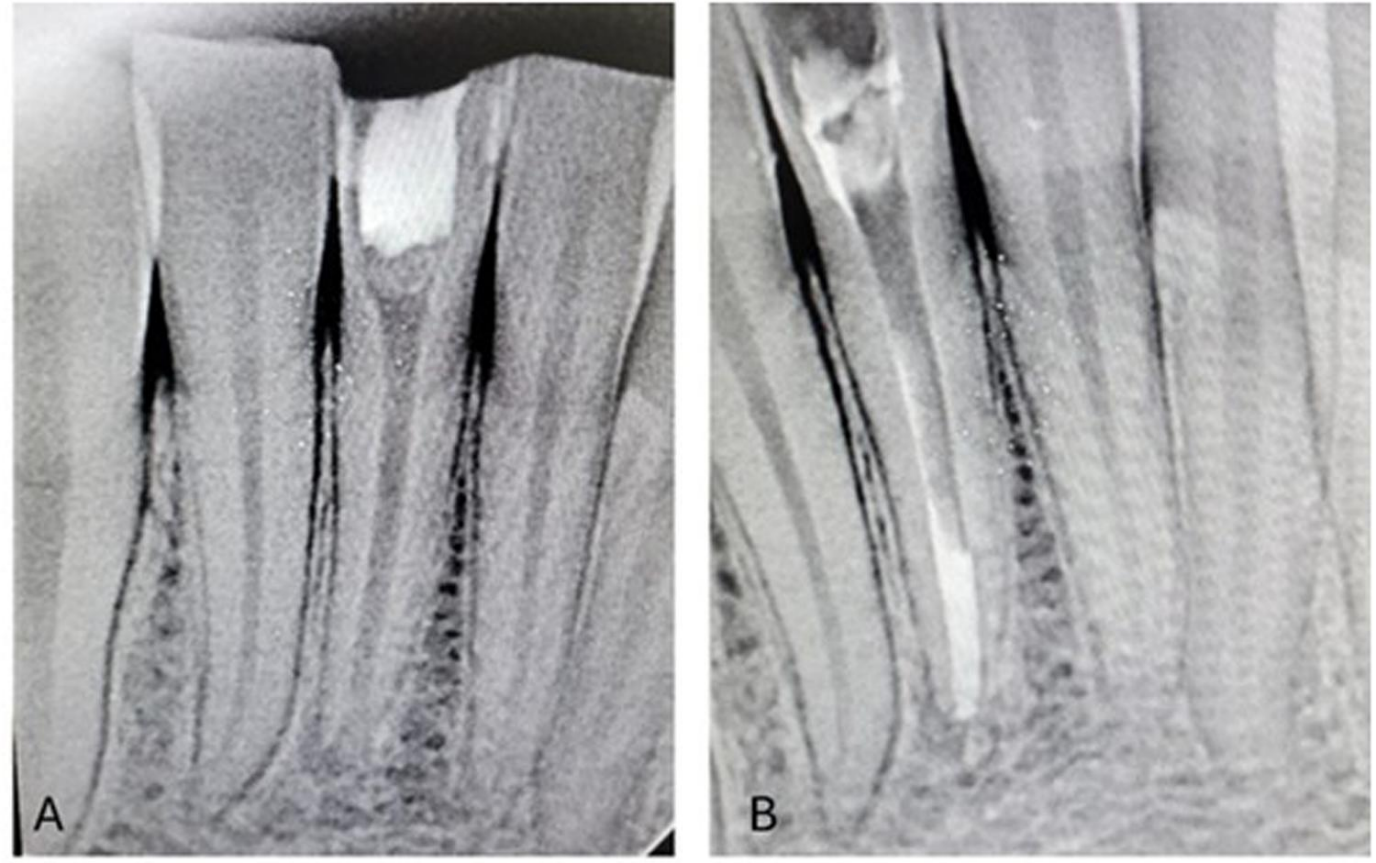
Postop IOPA radiographic representation of regenerative endodontic procedures with the use of biodentine. (A) Placement of amniotic membrane, (B) Application of Biodentine as apical plug.
